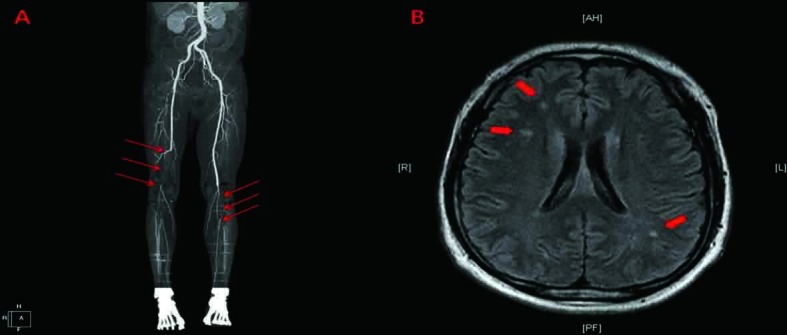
Computed tomography angiography and brain magnetic resonance imaging showed obstructions of right femoral and popliteal artery and left popliteal artery (A) and spotty ischemic brain lesions (B).
